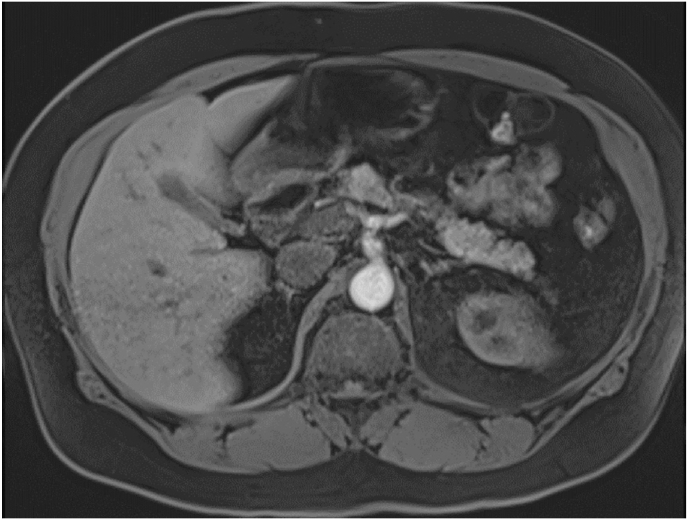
Follow up axial T1-weighted fat-saturated gadolinium-enhanced MR image showing regression of the mural and perivascular thickening previously seen in the celiac artery and its branches with the restoration of the lumen suggesting improvement of the underlying inflammatory process.
